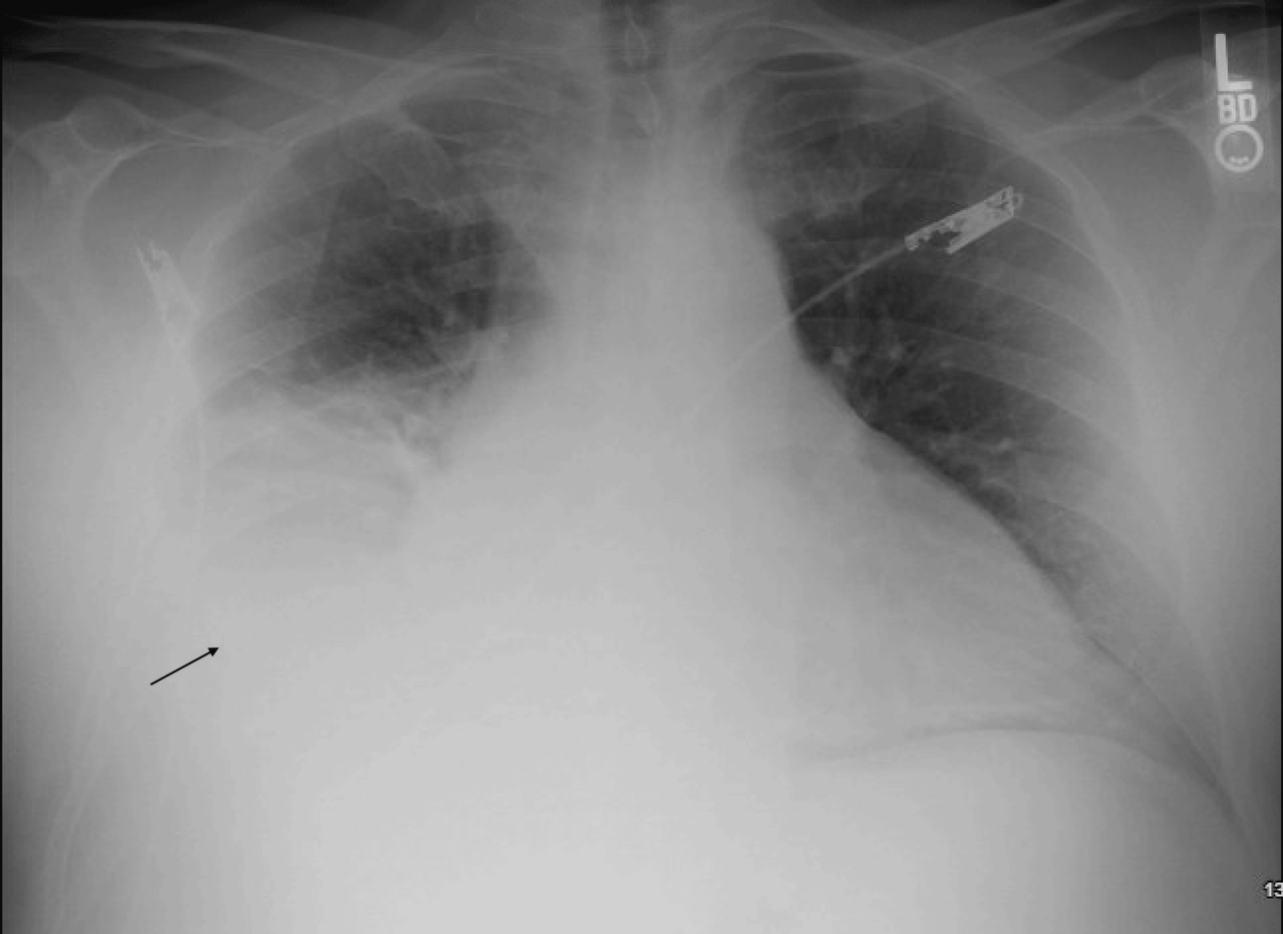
CXR (AP view): large right-sided pleural effusion (black arrow) CXR: Chest X-ray
